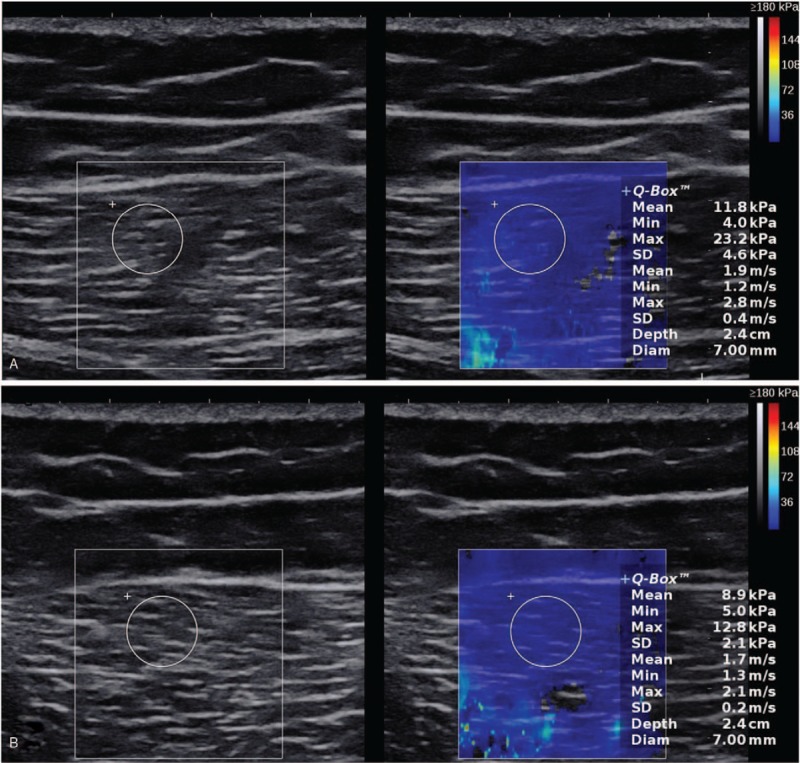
Ultrasound characteristics of nongluteal muscle contracture muscles. (A) Longitudinal view of the gluteus maximus muscle. (B) Transverse view of the gluteus maximus muscle.
